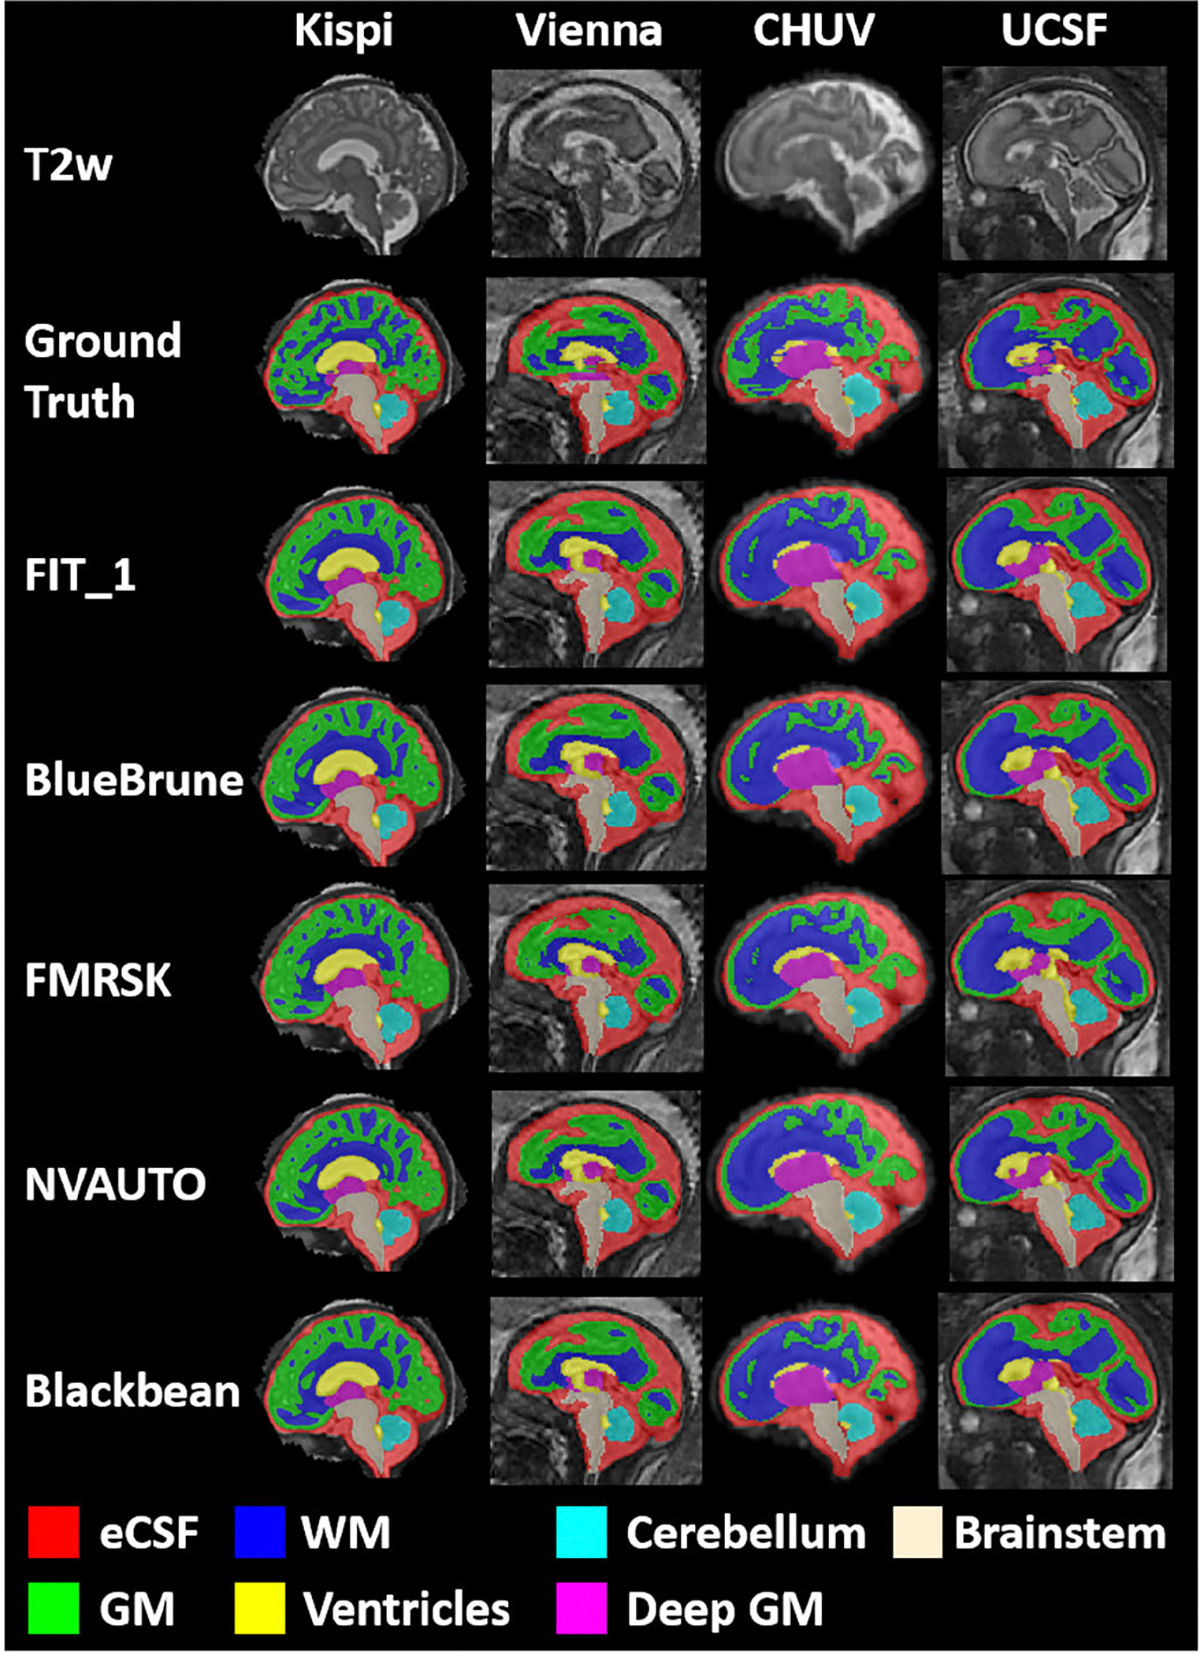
Examples of the automatic labels created by the top 5 teams for each of the four institutions (T2w: T2-weighted fetal brain reconstruction; eCSF: external Cerebrospinal Fluid; GM: Grey Matter; WM: White Matter).
